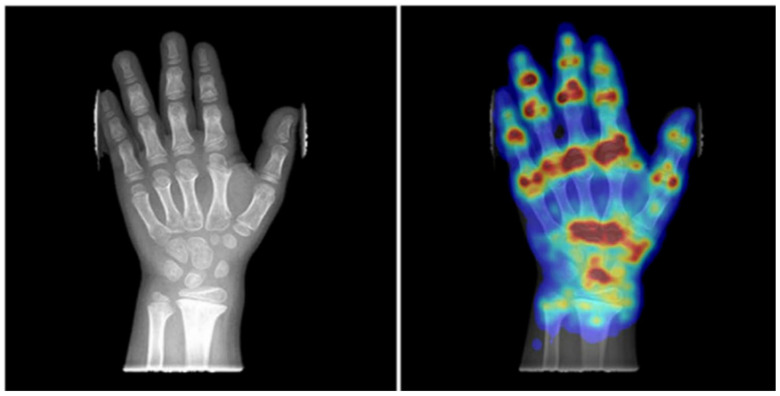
Radiograph and corresponding attention map produced by the hand bone age DL model for a 7-year-old girl with achondroplasia [26].
